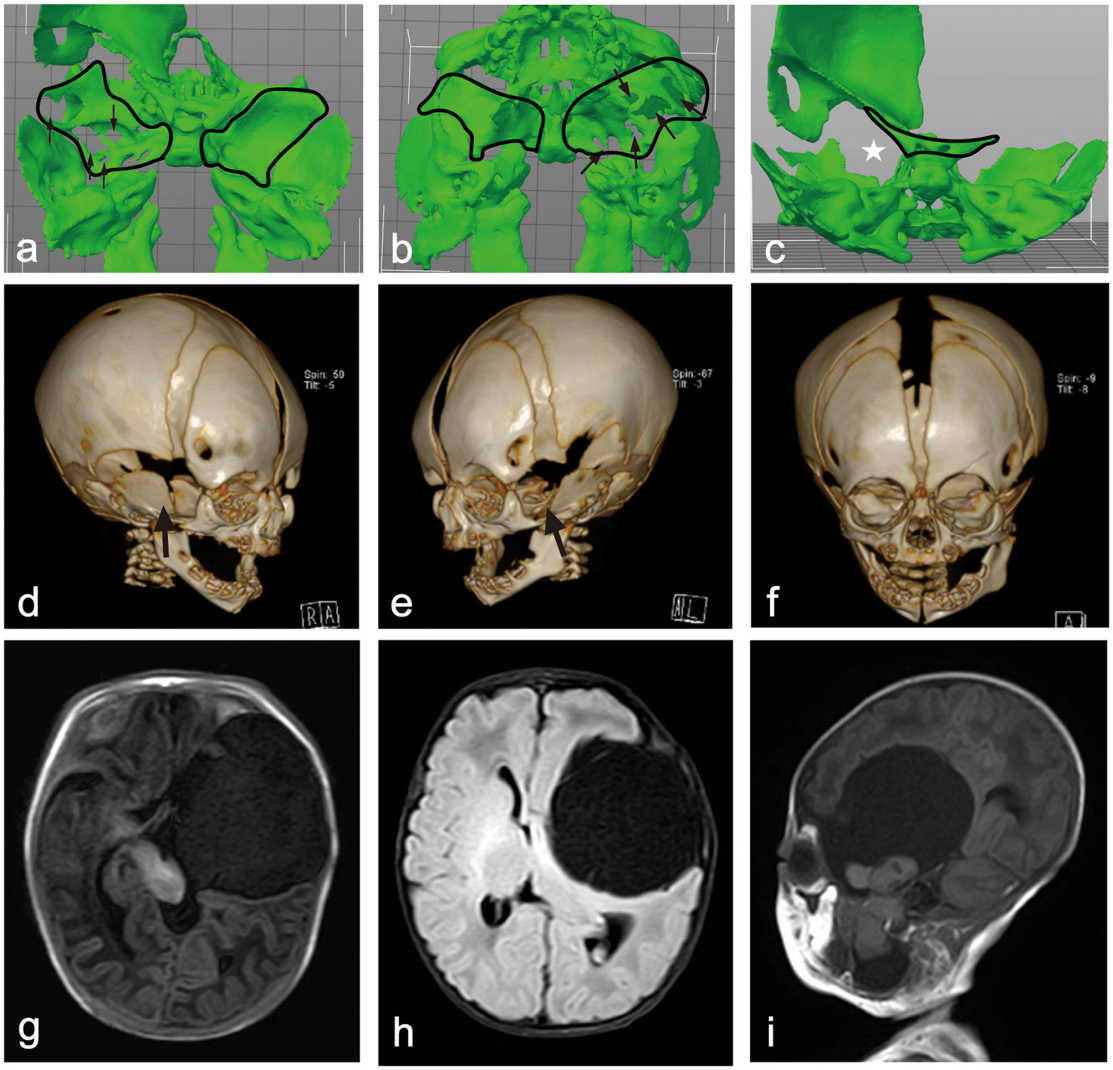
(a, b) A 3D model of the skull base above and below view showing multiple defects in the left greater wing of the sphenoid. (c) A 3-D model of skull base rear view showing upward of the lesser wing of sphenoid with corresponding left superior orbital fissure was enlarged. (d, e) A 3-D CT image of the skull's right and left frontal view shows the right GWS ossified well and a defect on the outside of the left. (f) A 3-D CT image of the skull's frontal view shows that the left orbit was enlarged compared to the right side, and the left mandibular head, neck, ramus, and angle were shifted laterally. (g–i) Axial T1WI, FLAIR, and sagittal T1WI images show a CSF signal intensity cystic lesion in the left middle cranial fossa and an irregular lesion with brain tissue signal intensity with it. These lesions herniated into the mandibular fossa through the left GWS. The brain tissue and ventricles around the lesion were compressed and displaced, and the midline was also displaced to the right. The left eyeball protruded forward from the contralateral side.
